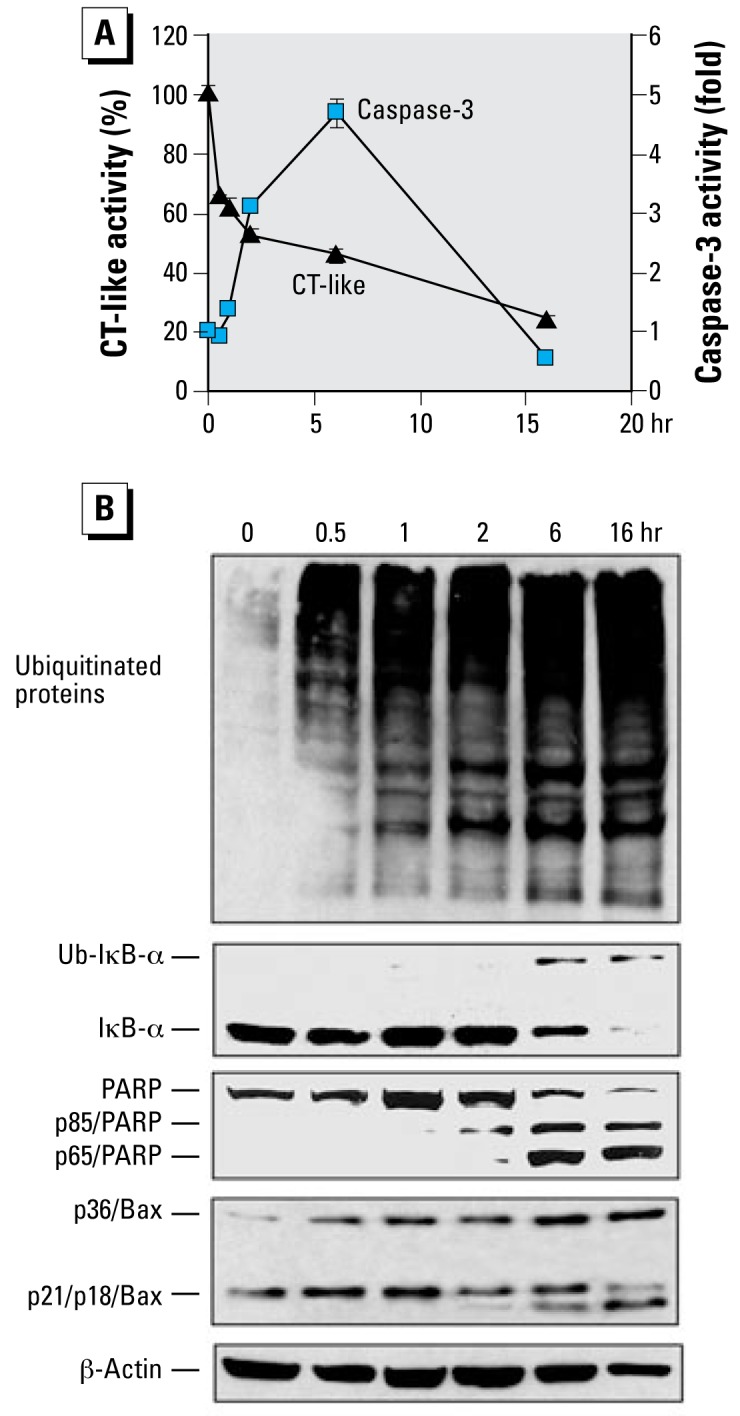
Kinetic studies on proteasome inhibition and cell death induction by TPT. (*A*) Exponentially grown MDA-MB-231 cells (0 hr) were exposed to 5 μmol/L of TPT for the indicated hr, and proteasome CT-like (triangles) and caspase-3 (squares) activities were measured. Data are mean ± SD of three experiments. (*B*) Western blot analysis of samples from the same experiment using specific antibodies to ubiquitin, IκB-α, PARP, Bax, and β-actin.
